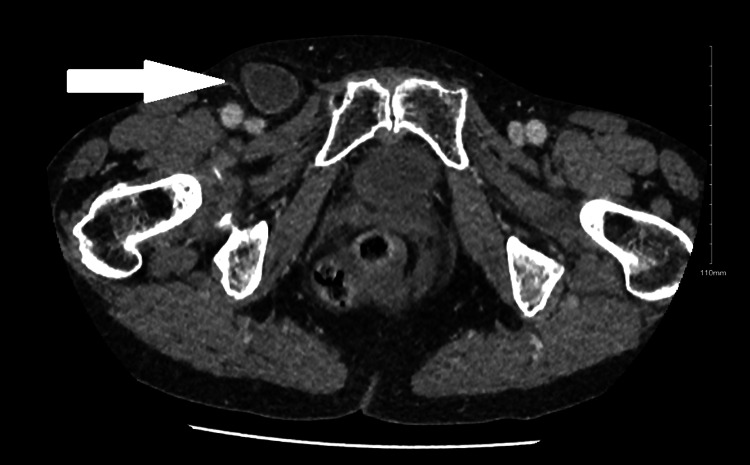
Axial view of right femoral hernia on computed tomography of the abdomen and pelvis.
